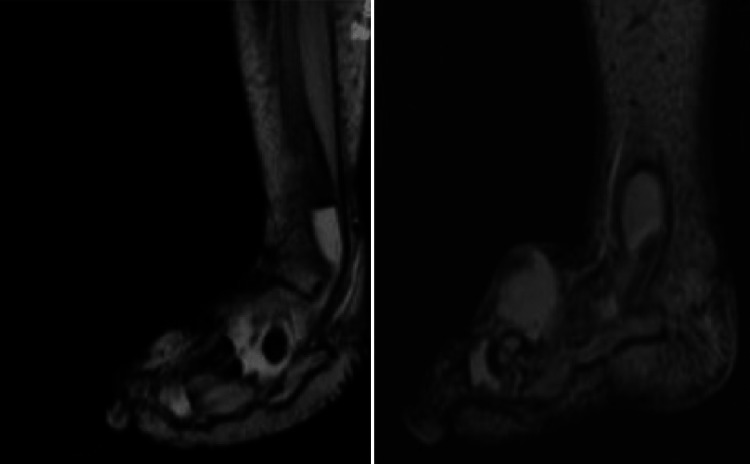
MRI showing the intramedullary expansion of the 1st metatarsal bone with cortical thinning and the presence of periosteal reaction Soft tissue around the metatarsal showed some signal changes with extension dorsomedially that caused bulging [14].
